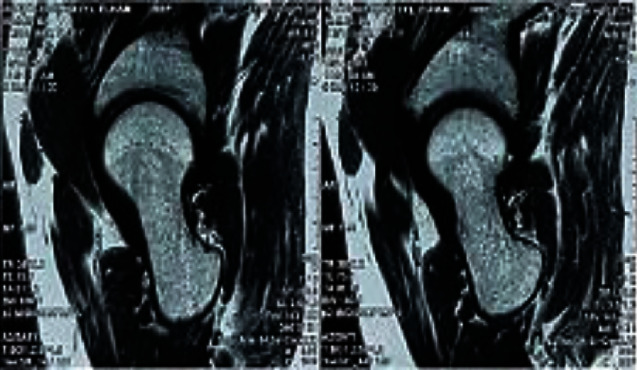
T2-weighted MRI of the hip showing normal condition of the hip cleared from any abnormal lesion postoperatively.
